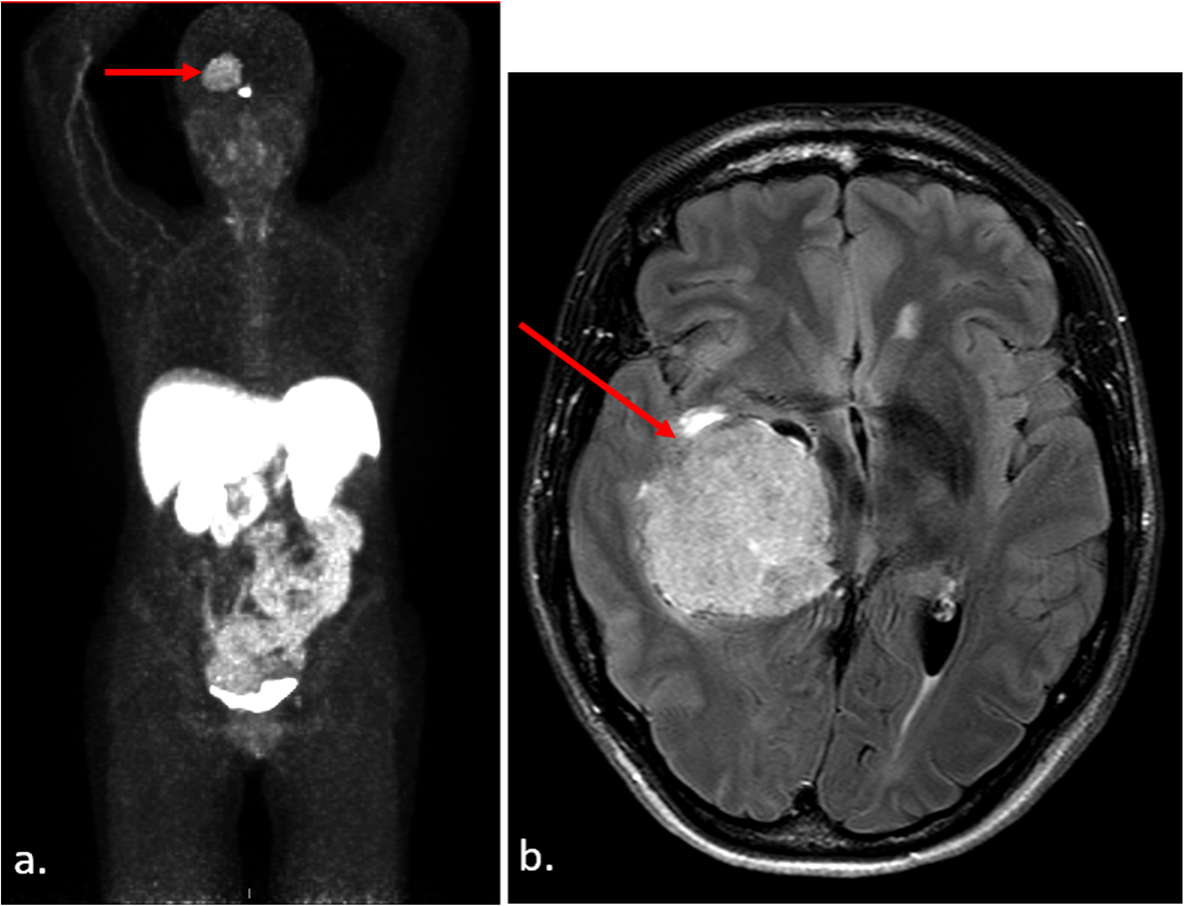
^68^Gallium-DOTATATE PET/CT study revealing a right-sided, avid intracranial mass. a. Planar whole-body maximum-intensity projection, with red arrow identifying the intracranial mass. b. Fluid-attenuated inversion recovery (FLAIR) post-contrast brain MRI demonstrating a 5.3 × 3.3 × 4.3 cm enhancing intraventricular mass (identified with red arrow) in the temporal horn of the right ventricle, which was associated with near-complete compression of the right lateral ventricle and 6 mm midline shift at the level of the foramen of Monro
